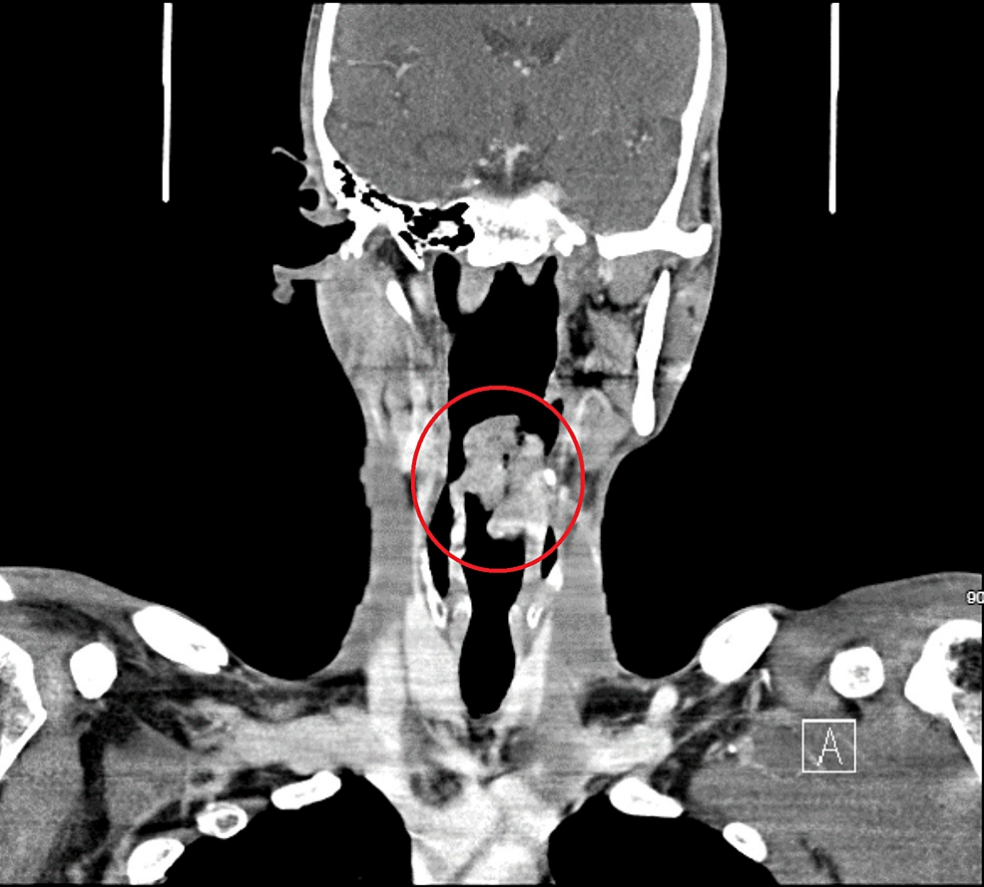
Computed tomography of the soft-tissue neck with contrast coronal section (lesion measuring 4.2 × 3.7 × 6.7 cm (AP, TR, SI) in the circle). AP: anteroposterior; TR: transverse; SI: superior to inferior
